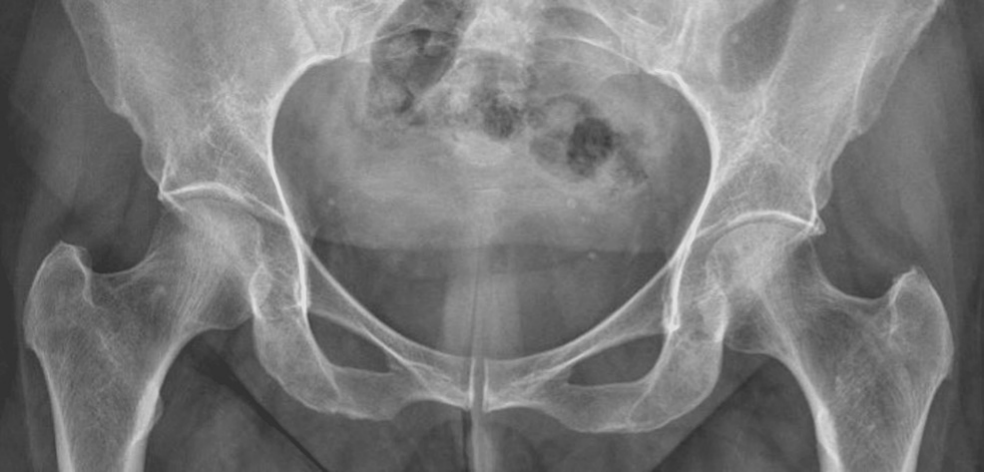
Plain radiograph of bilateral hip joints. The image shows intact joint spaces and no evidence of osteopenia.
